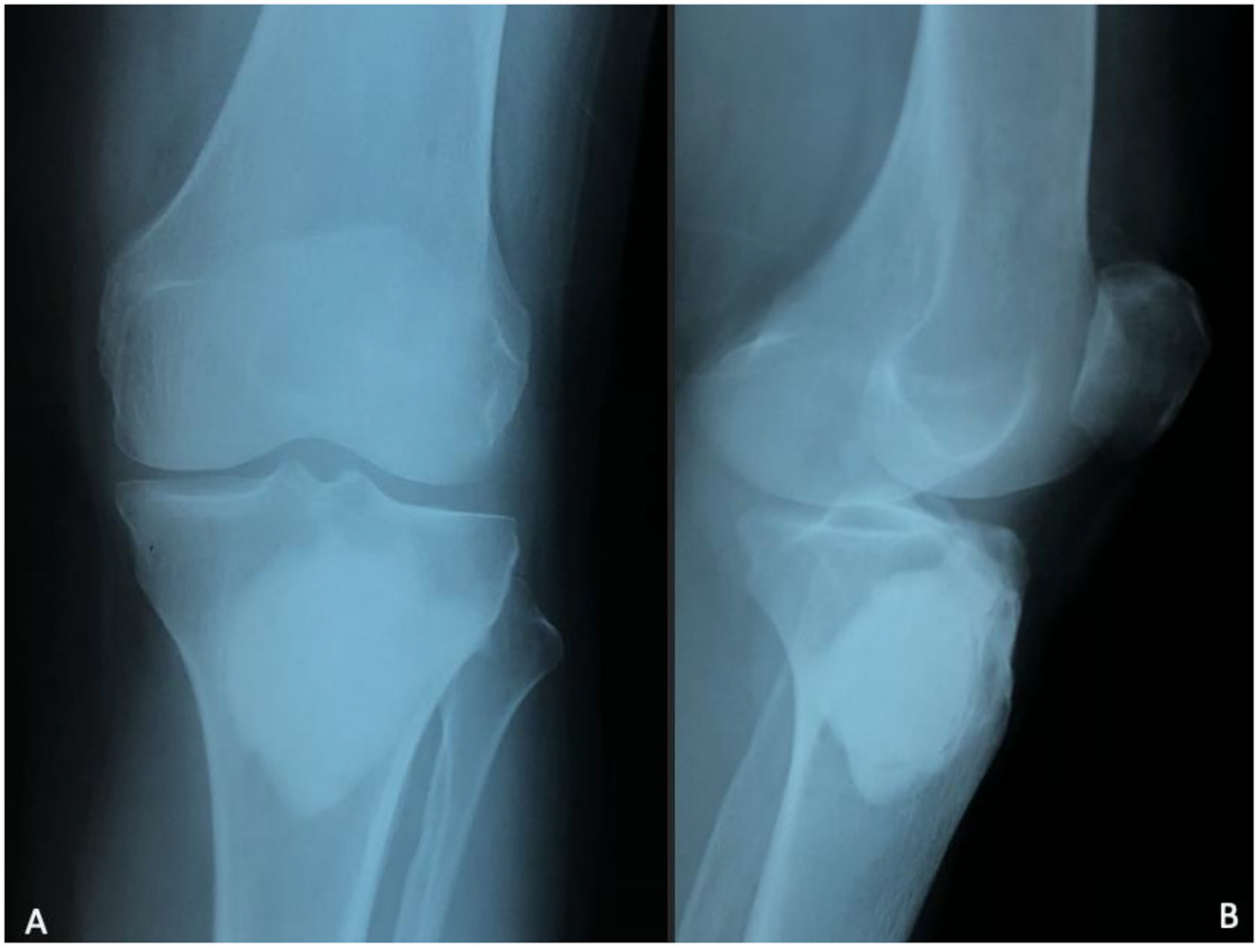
Follow-up radiographs at the last evaluation show no recurrence of the lytic process, with the bone cement in place at the proximal end of the tibia.
